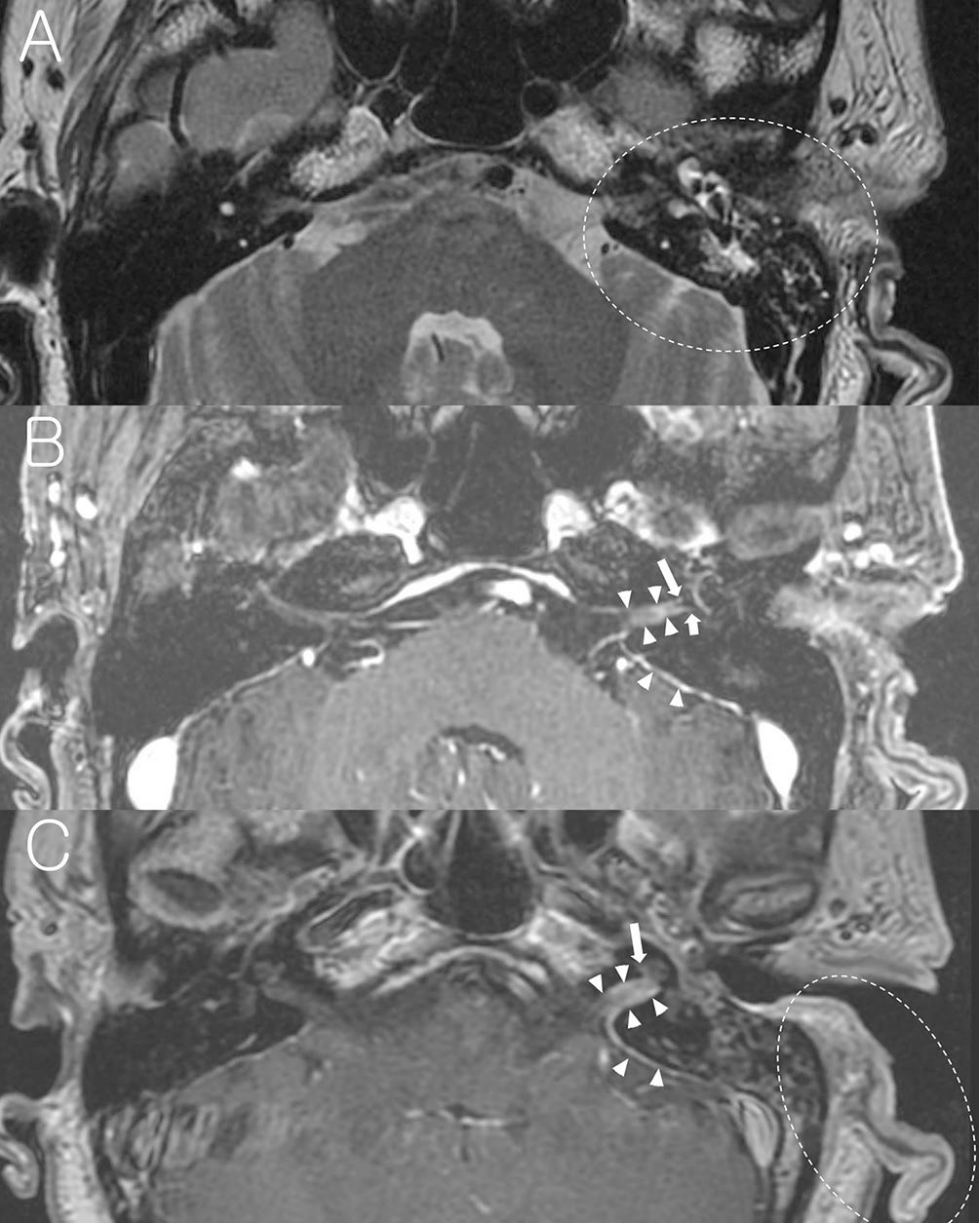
A representative case of Ramsay Hunt syndrome with mastoid effusion on the left side. (A) Non-contrast axial T2-weighted image at the level of IAC and mastoid air cells shows high signal intensity from the fluid in the left mastoid suggesting mastoid effusion (dotted circle). (B) Post-contrast axial T1-weighted image at the Bill’s bar shows enhancement of the distal canalicular and labyrinthine segment of the left facial nerve (long arrow), and superior vestibular nerve (short arrow). The adjacent dura in fundic area of the left IAC and left posterior petrous ridge are also enhanced (arrowheads). (C) Post-contrast axial T1-weighted image at the level of IAC demonstrates mild enhancement of basal turn of the left cochlea and diffuse dural enhancement along the whole length of the left IAC and left posterior petrous ridge (arrow heads). Left auricle is thickened and enhanced (dotted circle) as compared with right side.
